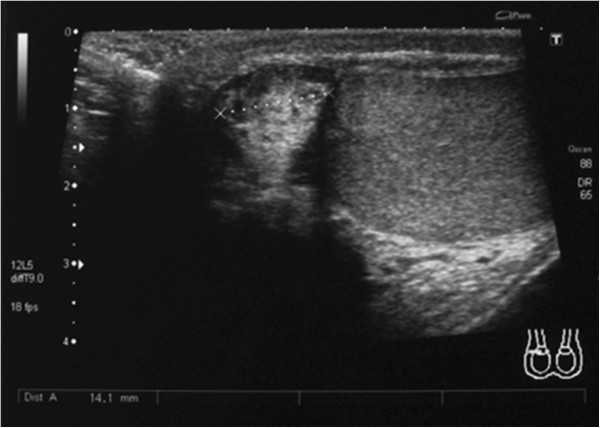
Ultrasound scan of the right hemiscrotum showing a nonhomogeneous 1.5 × 2 cm mass with no evidence of tissue infiltration or pathological lymph nodes.
